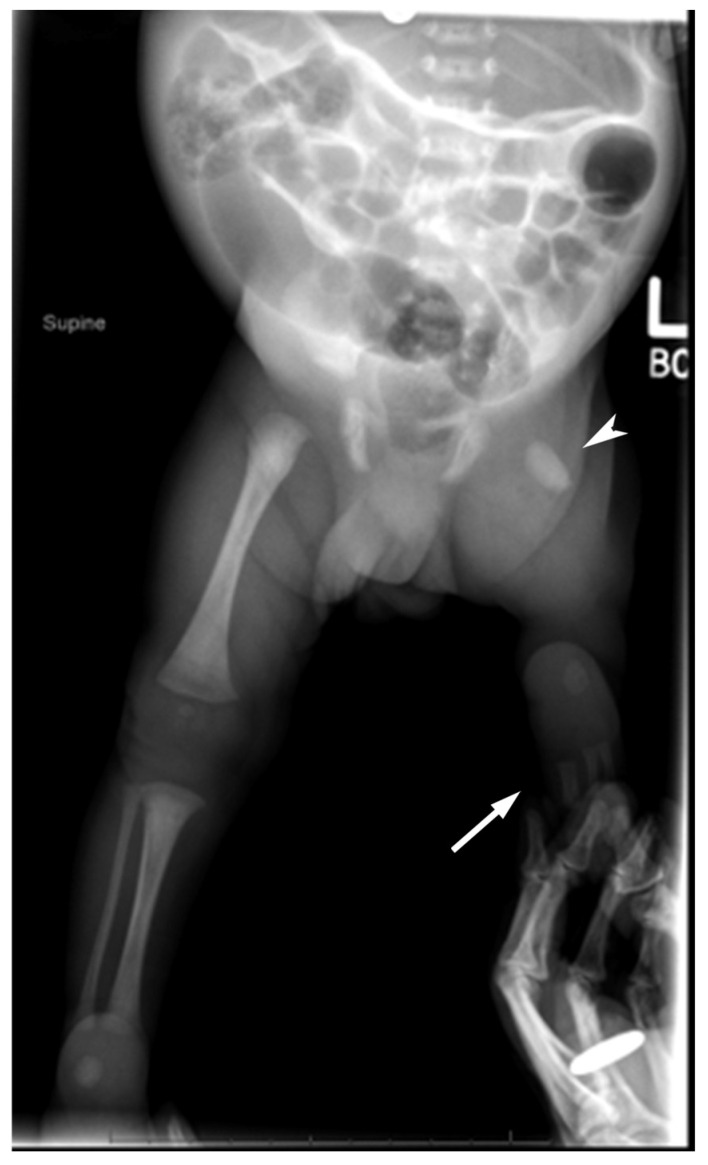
AP bilateral lower extremity radiograph at 6 months of age shows a diminutive left femur (arrowhead), no ossification of the tibia or fibula (white arrow), and 3 digital rays in the left foot.
